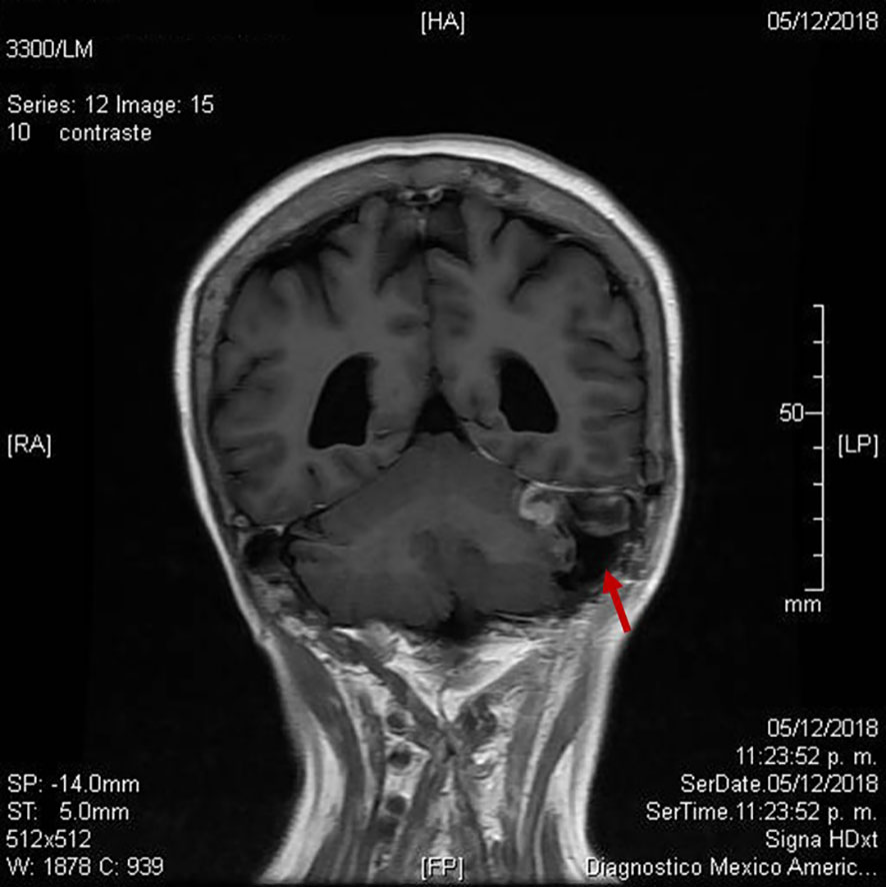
Postoperative magnetic resonance imaging following retrosigmoid craniotomy (red arrow).
